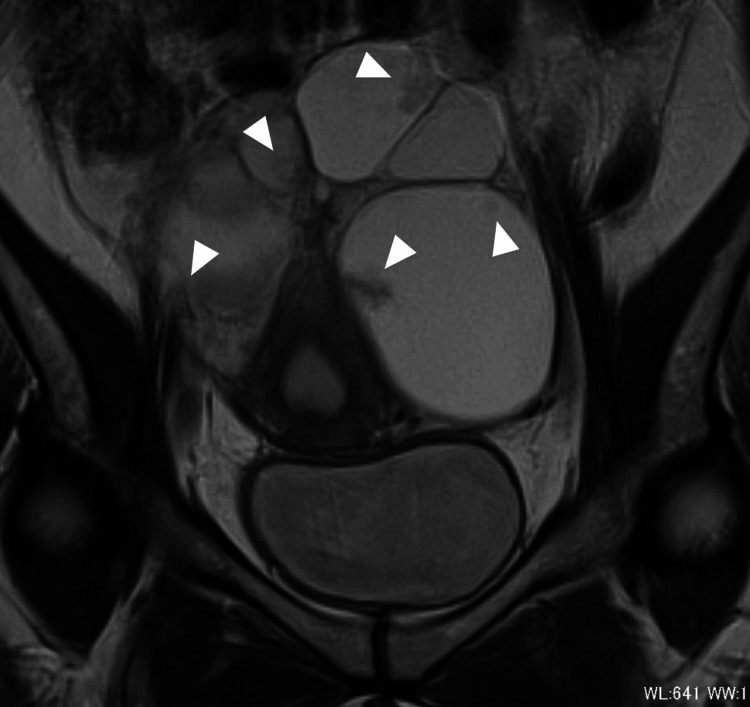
Preoperative T2-weighted magnetic resonance imaging scan (coronal section) showing bilateral multiloculated ovarian tumors with intracystic papillary projection (arrowheads).
